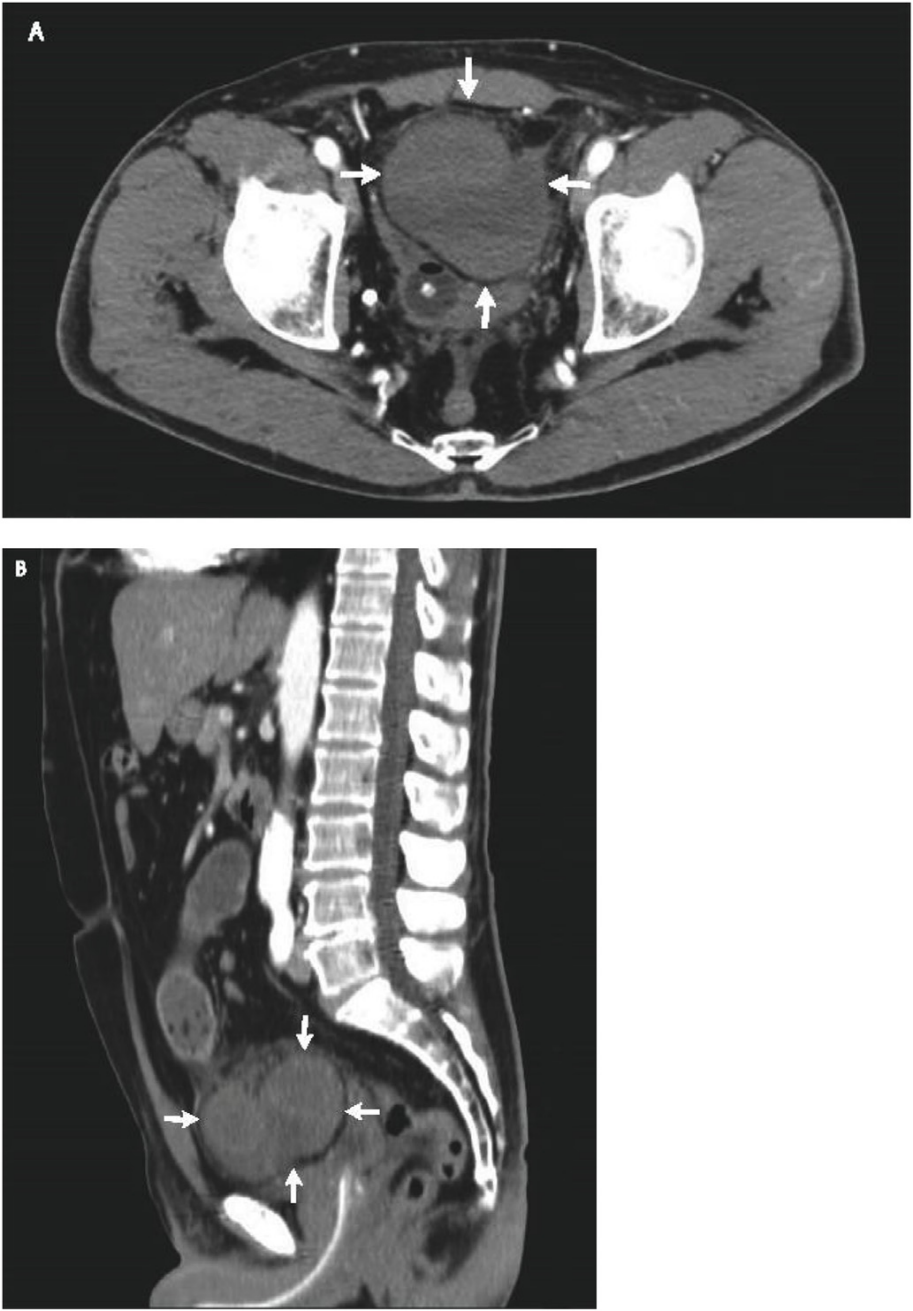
CT scan showed saclike mass of clustered dilated bowel loops (arrows) descending downward anterior and laterally to the bladder, compressing it. Sagittal (A) and coronal (B) sections.
